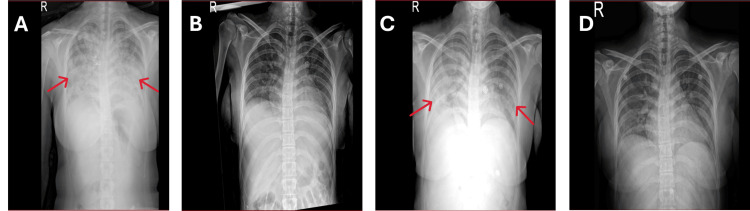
Serial chest radiographs showing the clinical course. (A) Initial presentation with diffuse bilateral airspace opacities. (B) Interval improvement following initial immunosuppressive therapy. (C) Relapse with recurrent bilateral infiltrates. (D) Partial radiographic resolution after treatment with corticosteroids and rituximab.
